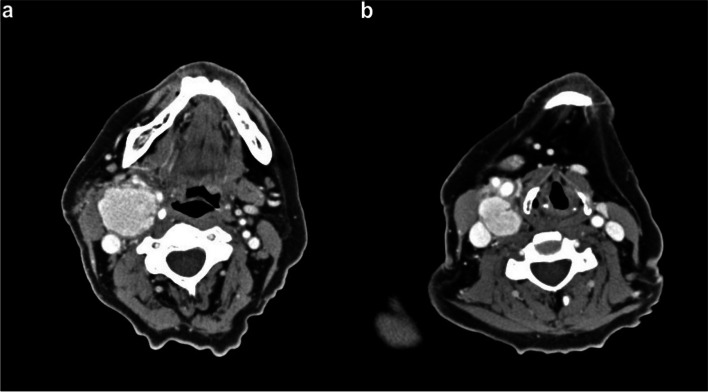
CT scan of a tumor in patient 1. a and b Horizontal CT scans of a well-defined tumor with robust, homogeneous contrast enhancement, measuring 34 × 31 mm in the transverse plane and approximately 60 mm in the craniocaudal dimension on the right side of the neck
